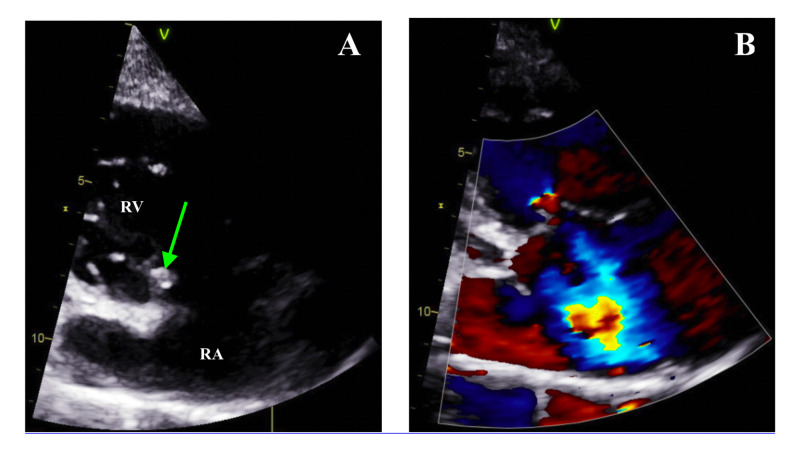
RV inflow view on transthoracic echocardiogram (from parasternal long axis view): (A) large vegetation (green arrow) measuring 21 x 5 mm on the tricuspid valve, (B) severe tricuspid regurgitation, as seen by the blue jet RA: right atrium, RV: right ventricle
